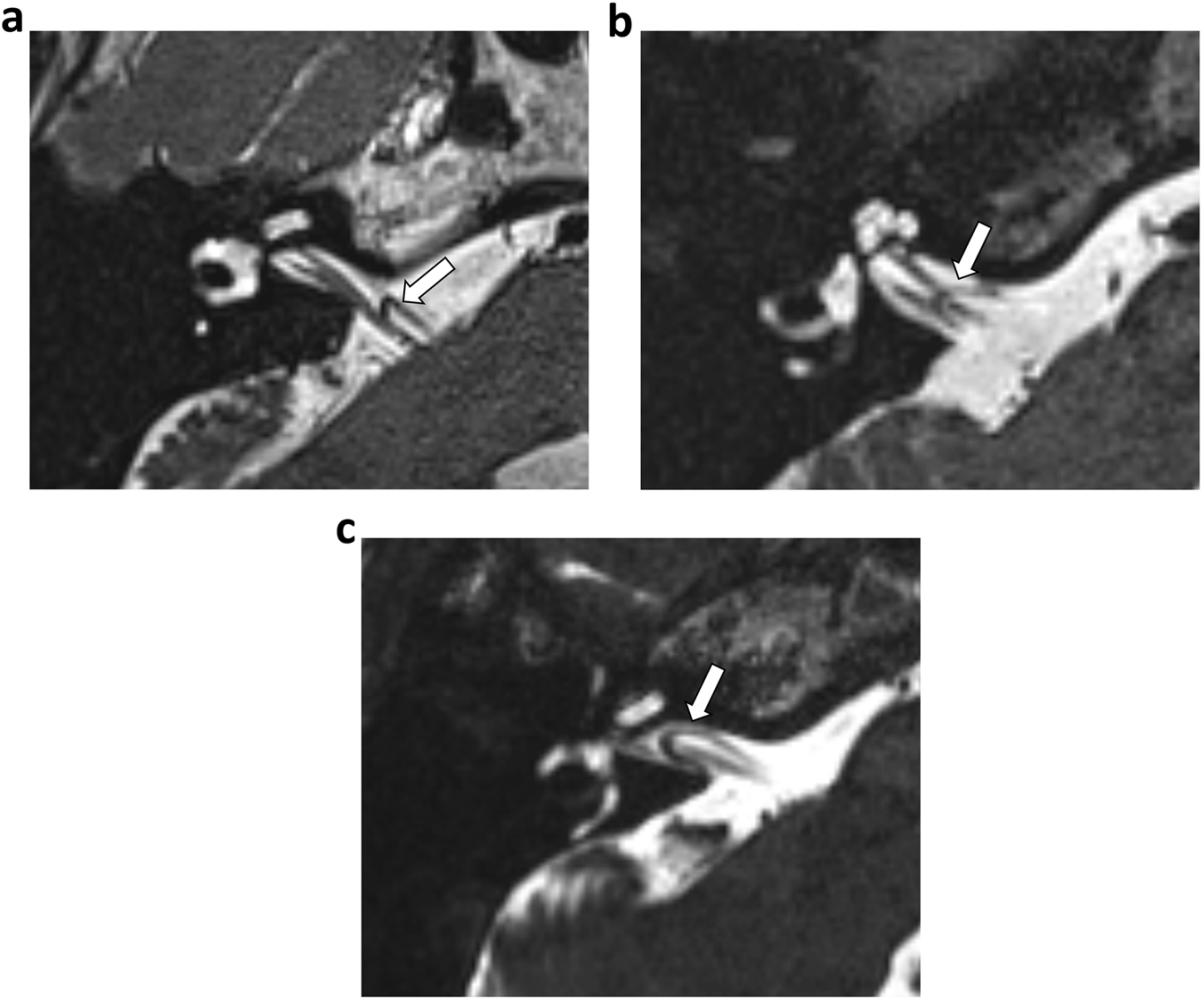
Image analysis for Chavda classification. Unenhanced axial T2w CISS images demonstrate the Chavda classification. VLs are indicated by white arrows. a Chavda I, VL located only in the CPA but not entering the IAM. b Chavda II, VL entering but not extending greater than 50% of the length of the IAM. c Chavda III, VL extending greater than 50% of the length of the IAM. CISS, constructive interference in steady state; IAM, internal auditory meatus; T2w, T2-weighted; VL, vascular loop
